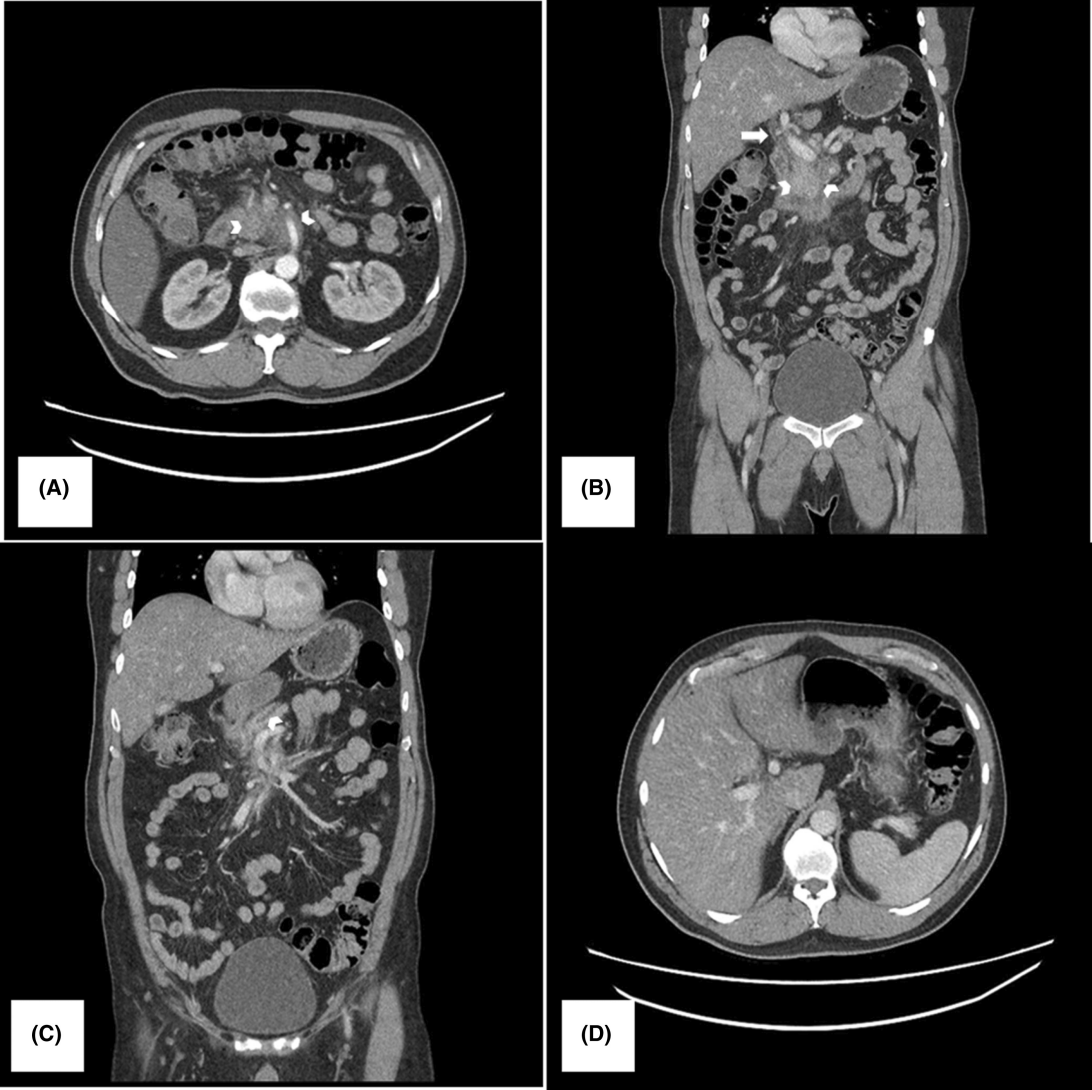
52‐year‐old male whose CT abdomen examination was requested with the indication “hepatic steatorrhea and left parapelvic cyst”. Serum IgG4 levels elevated to 1130 mg/L. (A) Abdominal contrast‐enhanced CT abdomen (CECT) axial section, showing a mildly enhancing soft tissue density mass involving the uncinate process of pancreas and also completely encasing superior mesenteric artery (white arrowhead). (B) Coronal section from CECT. Showing that mass is also encasing the pancreatic head, uncinate process and distal common bile duct (CBD) (white arrowhead). In addition to, there is the faint enhancement of the walls of CDB as well, one of the ancillary findings in IgG‐4‐related disease (white arrow). (C) Another coronal section of CECT at the level of the pancreatic duct. There is borderline dilatation of the pancreatic duct (PD) measuring 2.8 mm (white arrowhead). However, there is no intrahepatic biliary dilatation. (D) Axial section from CECT, showing no intrahepatic biliary dilation despite distal mass.
